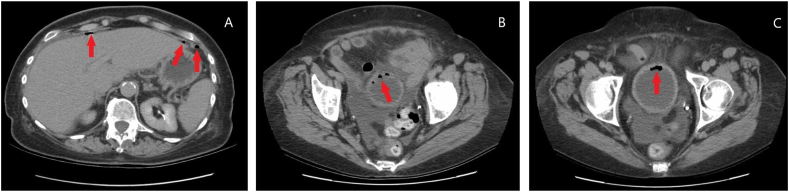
CT A/P with IV contrast. A: free air anterior to liver and stomach (denoted by red arrows). B (more superior): free air within the antero-superior aspect of the bladder wall (denoted by red arrow). C (more inferior): free air in the anterior bladder wall and lumen (denoted by red arrow), as well as free peritoneal air anterior to the bladder. (For interpretation of the references to colour in this figure legend, the reader is referred to the Web version of this article.)
